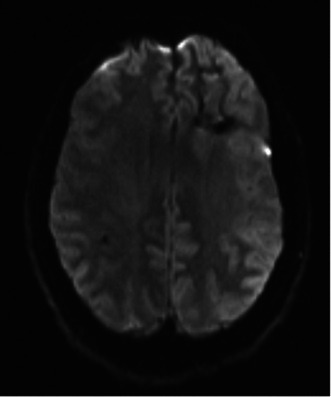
FIGURE 2 MRI brain demonstrated diffusion restriction in the bilateral basal ganglia with multifocal cortical ribboning, more prominent on the left.
